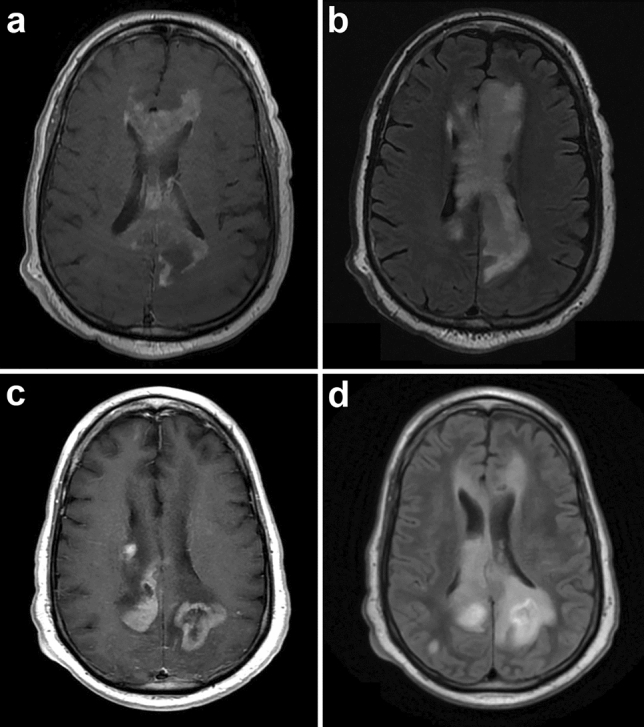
Initial axial, contrast-enhanced T1-weighted, magnetic resonance image demonstrating enlargement and amorphous enhancement involving the cingulate gyrus and corpus callosum, extending across the midline (a). There was also confluent periventricular white matter T2/FLAIR hyperintensity (b). Repeat imaging 3 months later showed multiple new contrast-enhancing masses (c) and progression of T2/FLAIR hyperintensity (d)
